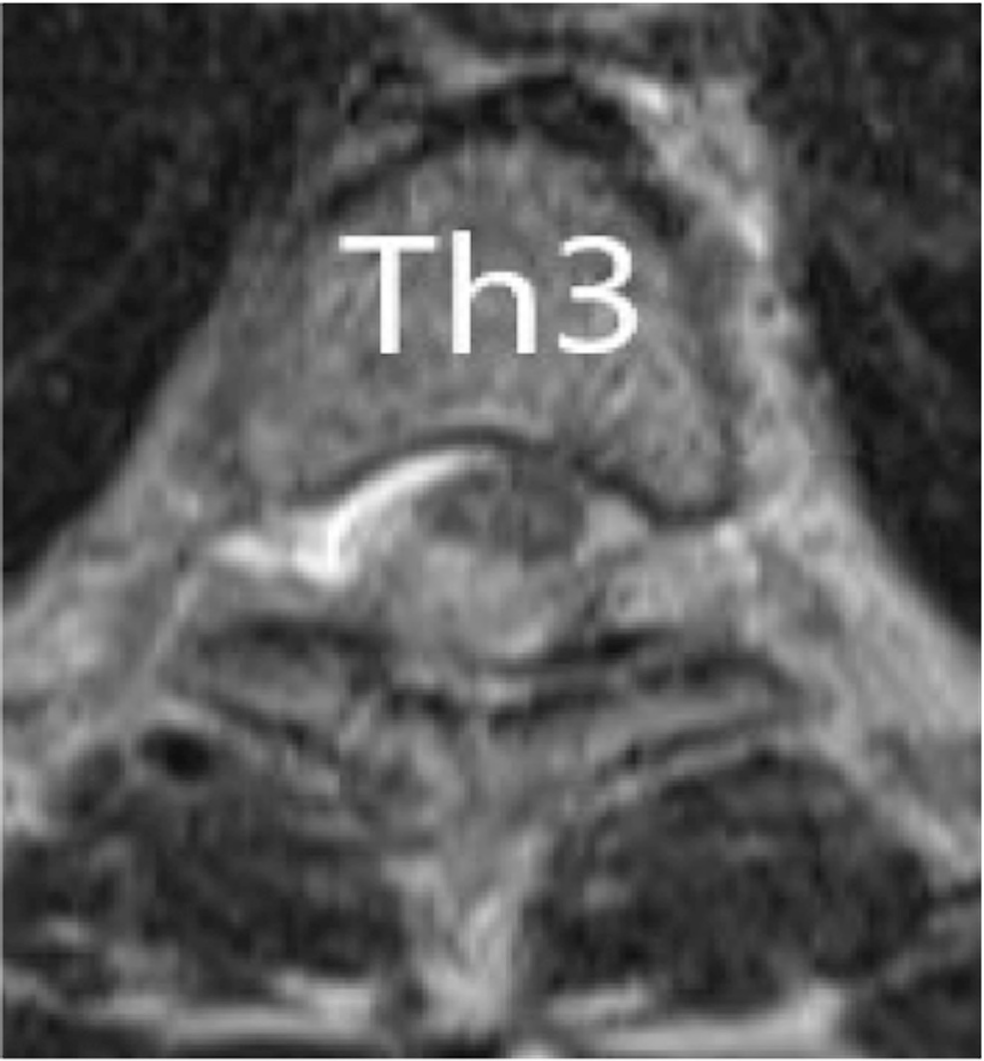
Axial T1-weighted MR images at the time of transfer The stenosis was located at the highest position of Th3, and the thoracic spinal cord was compressed to the left ventral side, with moderate to severe spinal cord compression.
